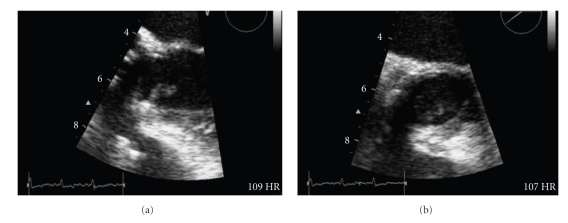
Transesophageal echocardiogram showing a pedunculated vegetation attached to the prosthetic aortic valve. (a) 0° horizontal midesophageal view. (b) 34° midesophageal short cardiac axis.
